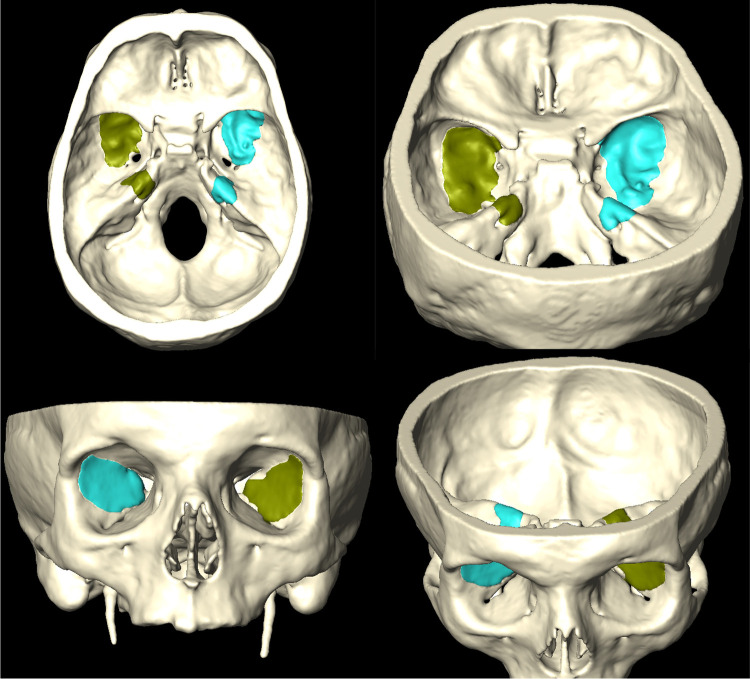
Amira software 3D reconstructions from postdissection CT scans, highlighting the amount of bone removal at the level of the middle cranial fossa and petrous apex.
